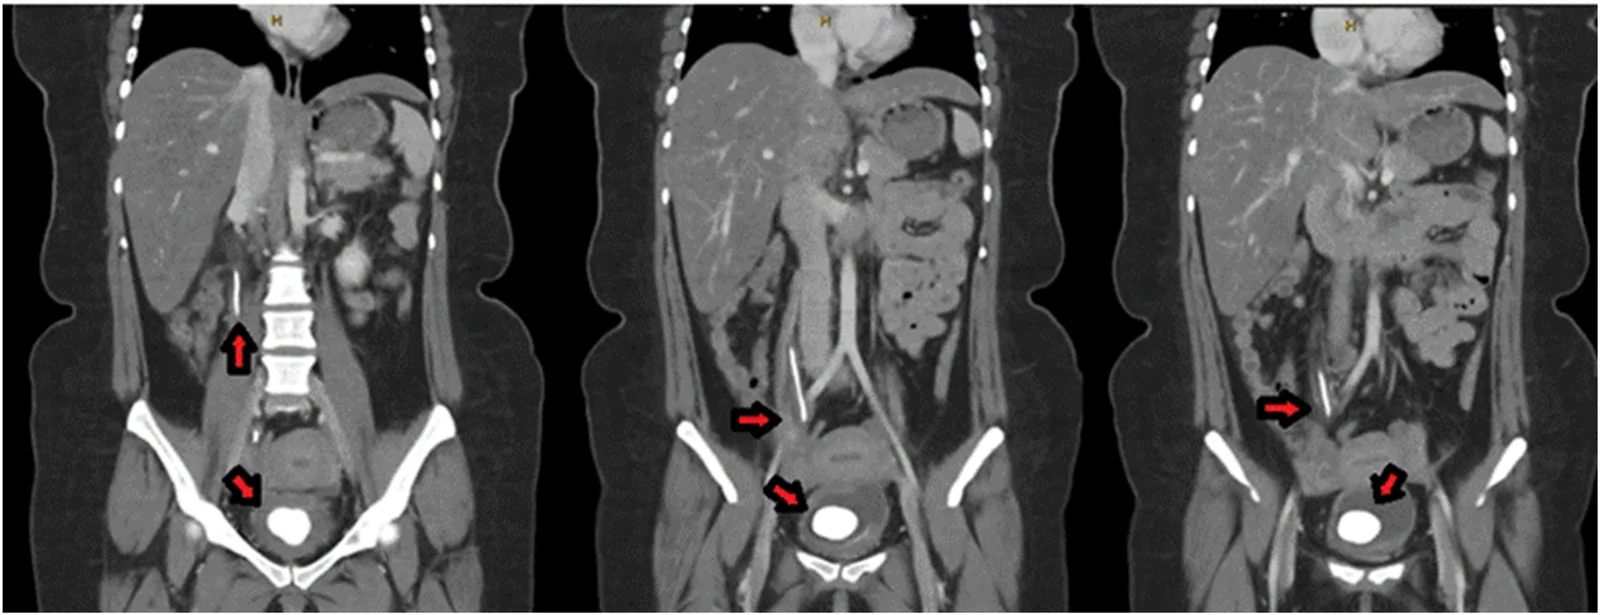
Coronal contrast-enhanced CT of the abdomen and pelvis demonstrating the calcified retained stent and associated distal calculi.
